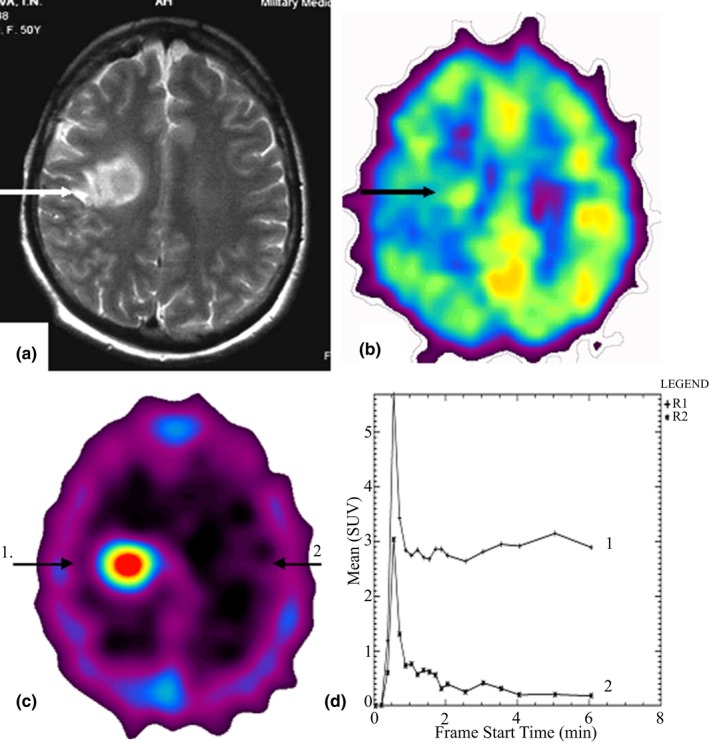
(a‐d), Female patient g. Diagnosis: glioblastoma multiforme recurrence growth of the right parietal lobe. (a) At MRI (T2‐weighted image), the tumor is well visible, and perifocal edema is defined. (b) ^18^F‐FDG PET image demonstrates heterogeneous unsharp mass with the high glycolytic rate glycolysis (UI = 1.0). The lesion is partially visible due to its location in the white matter as well as to edema and ischemization of the adjacent brain cortex. (c) ^82^Rb‐chloride PET image demonstrates a sharp homogeneous hypervascular focus with high radiopharmaceutical uptake in the tissue phase (UI = 17) (position 1). Contralateral brain cortex is shown in position 2. (d) Activity/Time curve of the lesion (1) shows that the tumor has the high vascularization degree (VD = 2.1). Vascular permeability is impaired. Within the tissue phase, high radiopharmaceutical uptake in tumor exceeds the radiopharmaceutical uptake in the contralateral brain cortex (2) in 17 times. A typical for malignant tumors slow monotonic radiopharmaceutical uptake in the tumor within the tissue phase is observed
